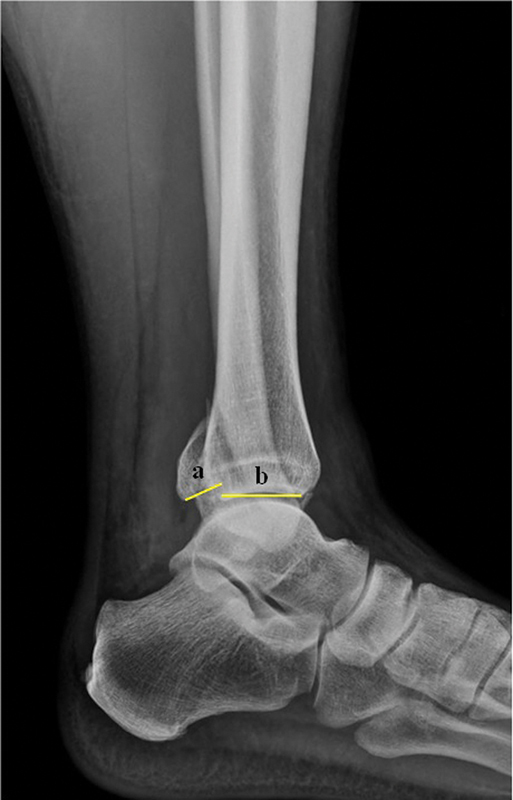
Measurement of the posterior malleolus fracture fragment size on a lateral radiograph. The proportion between the fracture and the entire articular surface was determined by the ratio (a)/(a + b).
